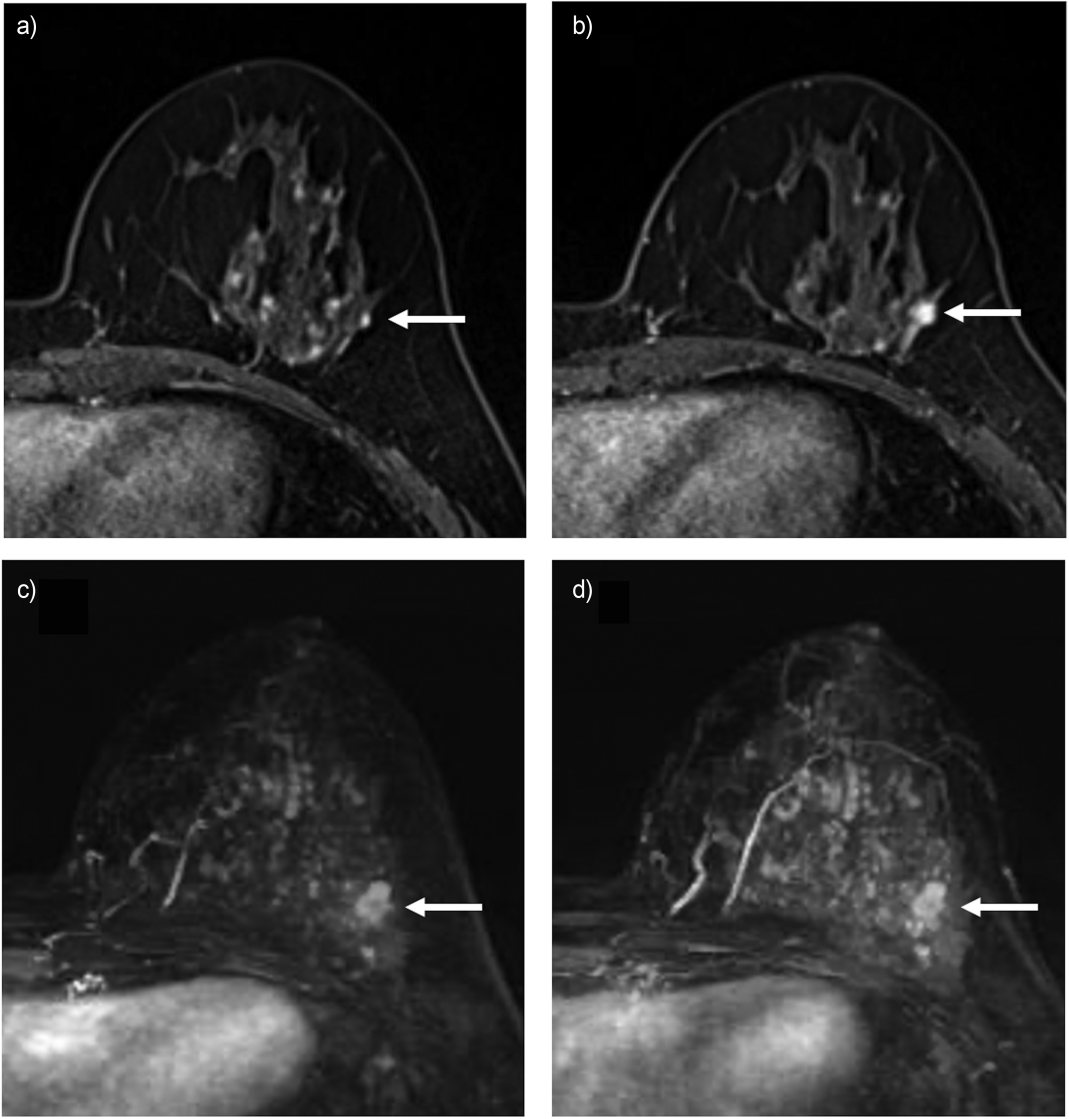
Woman with a pathogenic variant in *BRCA1* and a right breast-conserving surgery at her 30s. She started imaging screening using yearly MRIs after her operation. (a) and (b) are the 2nd phase of DCE-MRI. (a) A mammogram was assessed as “normal” 1 year before the lesion was suspected. (b) Due to the enlargement of an 8 mm enhancing lesion in the left outer lower quadrant region of the breast, the lesion was diagnosed as BI-RADS category 4A. The US followed up on the lesion, and 7 months later, a suspicious lesion was detected in the US and biopsied. The biopsy showed a luminal B type invasive ductal carcinoma. This was diagnosed 10 years after she got her first cancer. Then, preoperative MRI was performed. (c) is the 10th phase and (d) is the 20th phase of the UF DCE-MRI. The lesion is visible in the early phase of UF DCE-MRI with less BPE. The white arrows in each image indicate the lesion. BI-RADS, Breast Imaging Reporting and Data system; BPE, background parenchymal enhancement; DCE-MRI, dynamic contrast-enhanced MRI; US, ultrasound, UF, ultrafast.
